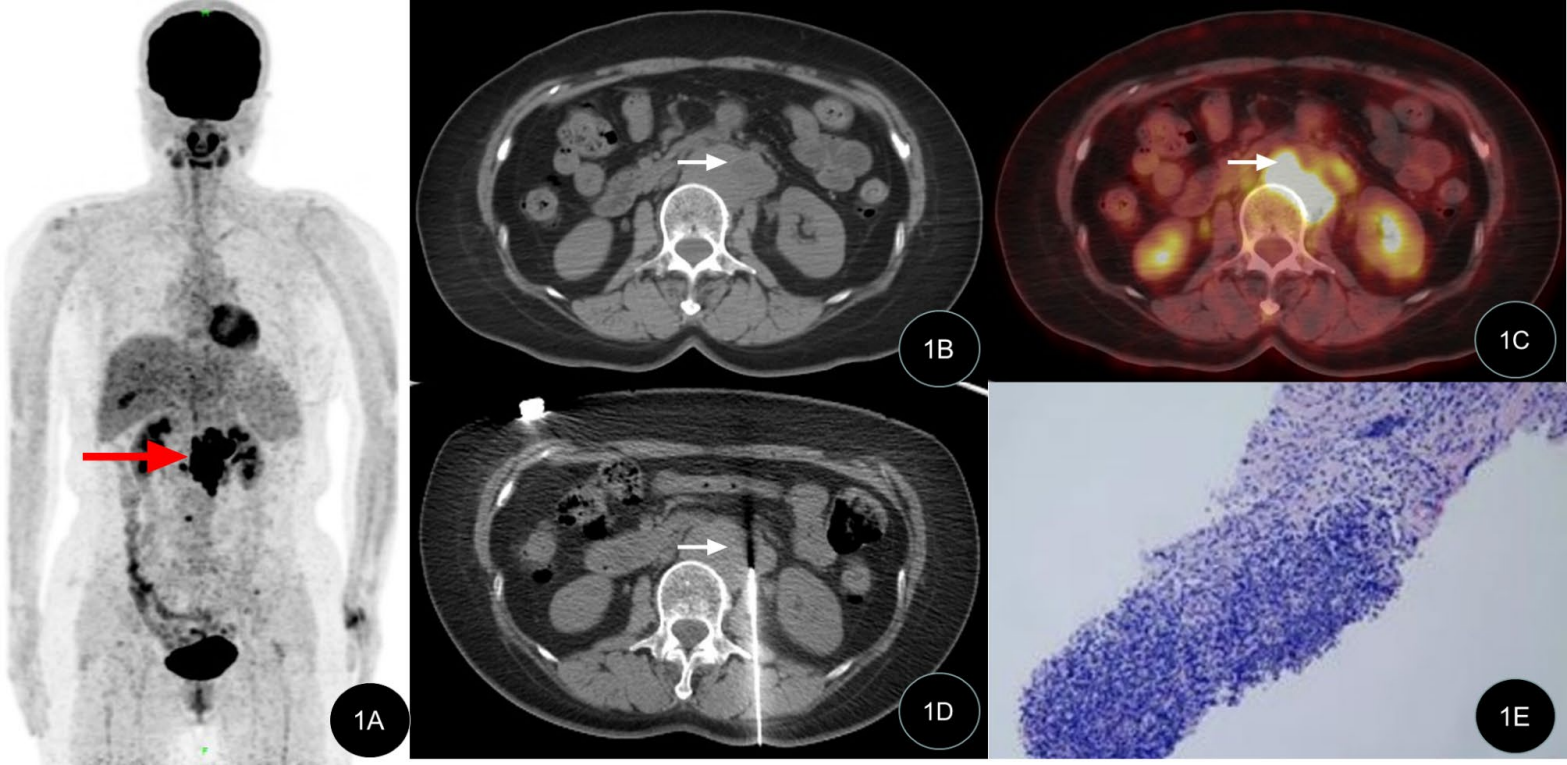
PET/CT-guided targeted retroperitoneal masses biopsy The patient (40-year-old male) was admitted due to abdominal pain. The PET/CT MIP image, PET/CT fusion CT image, puncture biopsy image, and biopsy pathological image are shown. 1 A. MIP image shows abnormal metabolism increase at the left side of the lumbar spine (indicated by red arrow); 1B, 1 C. PET/CT fusion CT image shows increased metabolism at the retroperitoneal mass; 1D. Puncture biopsy of the target area of the retroperitoneal mass under PET/CT guidance; 1E. Pathological examination shows highly invasive B-cell lymphoma (HE × 100)
